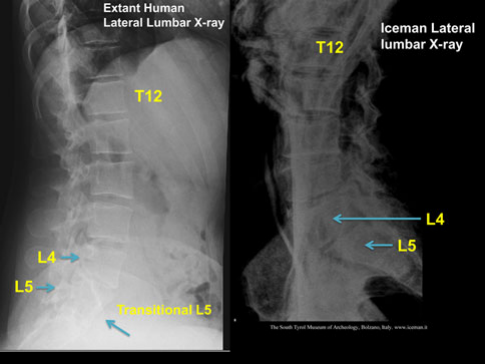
Iceman lateral lumbar X-rays in comparison to an extant human with the same configuration of a lumbar 5 transitional vertebra. The transitional L5 of the extant human and the Iceman are incorporated in the upper sacrum
